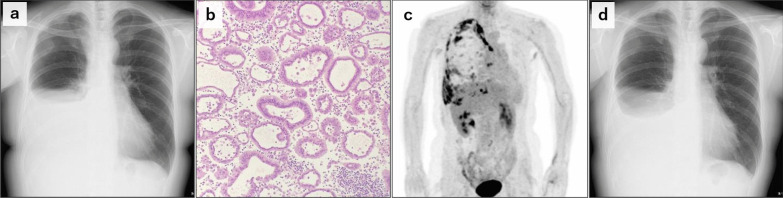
Cytology and imaging of carcinomatous pleurisy. a Chest X-ray at the time of recurrence diagnosis. b Tubular-forming metastatic breast cancer cells in pleural effusion. c Positron emission tomography scan at the time of recurrence diagnosis. d Chest X-ray 1 month after the initiation of targeted therapy
